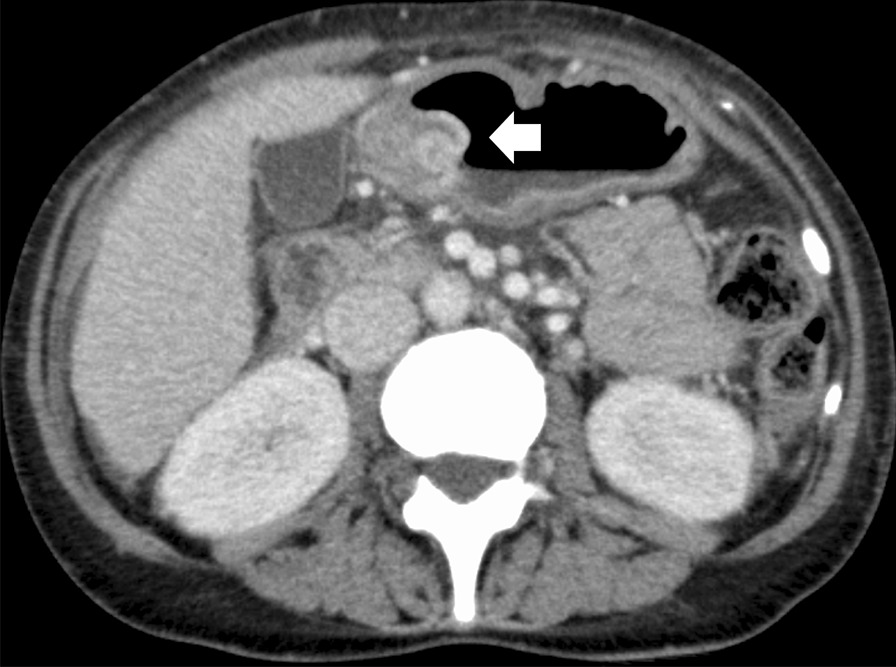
Computed tomography with contrast revealed non-specific thickening of the pylorus (arrow) with no clear signs of gastrointestinal bleeding
